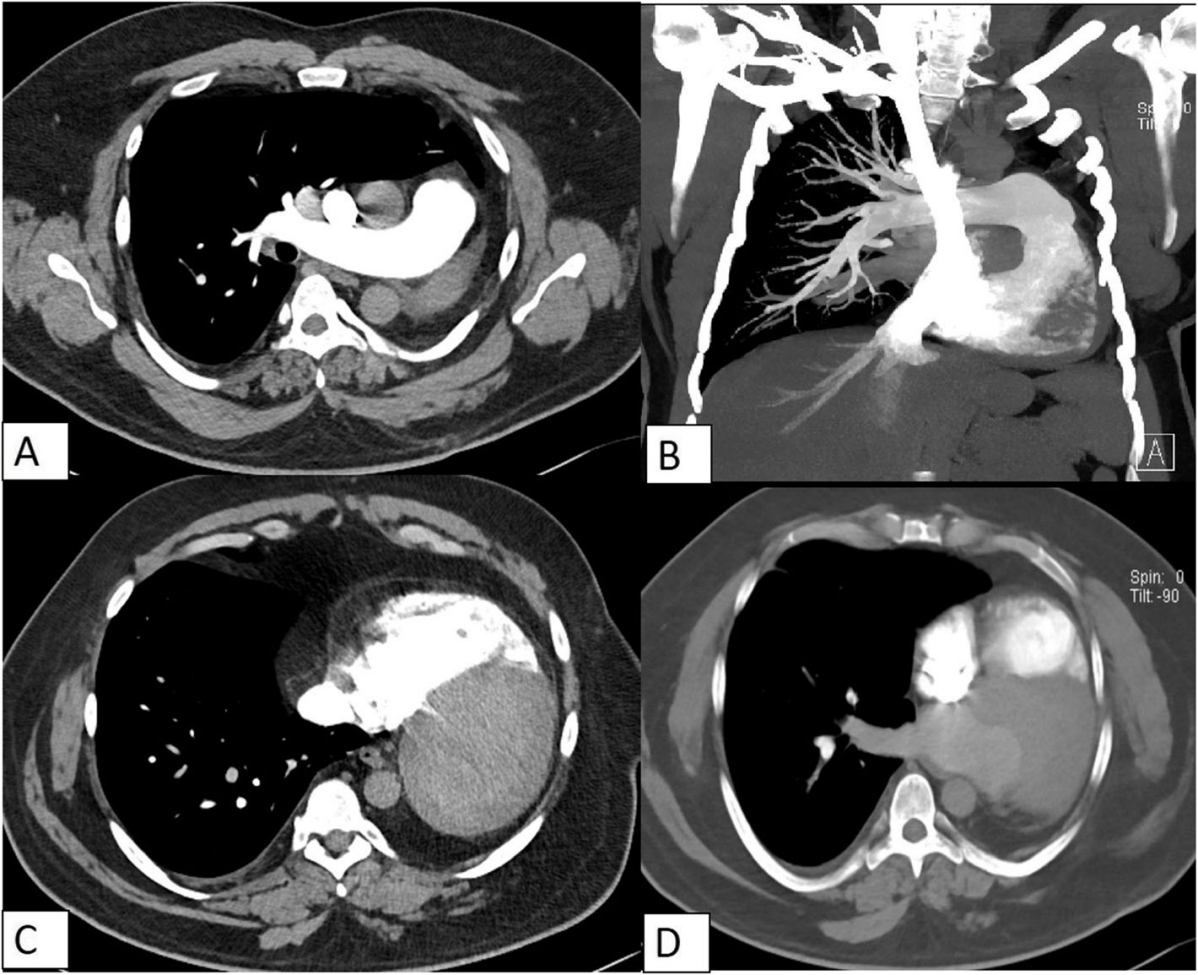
CT pulmonary angiogram showing continuation of patent main pulmonary artery into right pulmonary artery with absent left pulmonary artery (A, B). The heart is shifted into left hemithorax, lying flush with the chest wall with increased pericardial fat (C, D)
